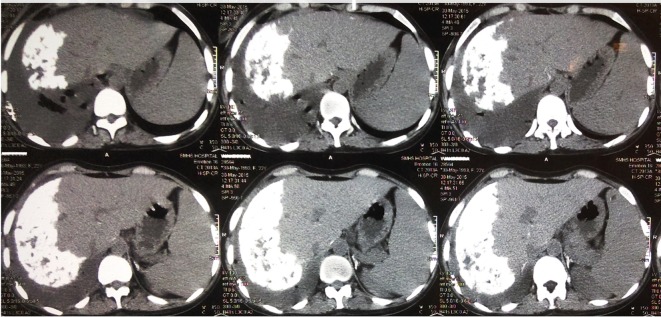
CT abdomen, axial view with serial images showing dense calcification in right hepatic lobe with pleural effusion.
